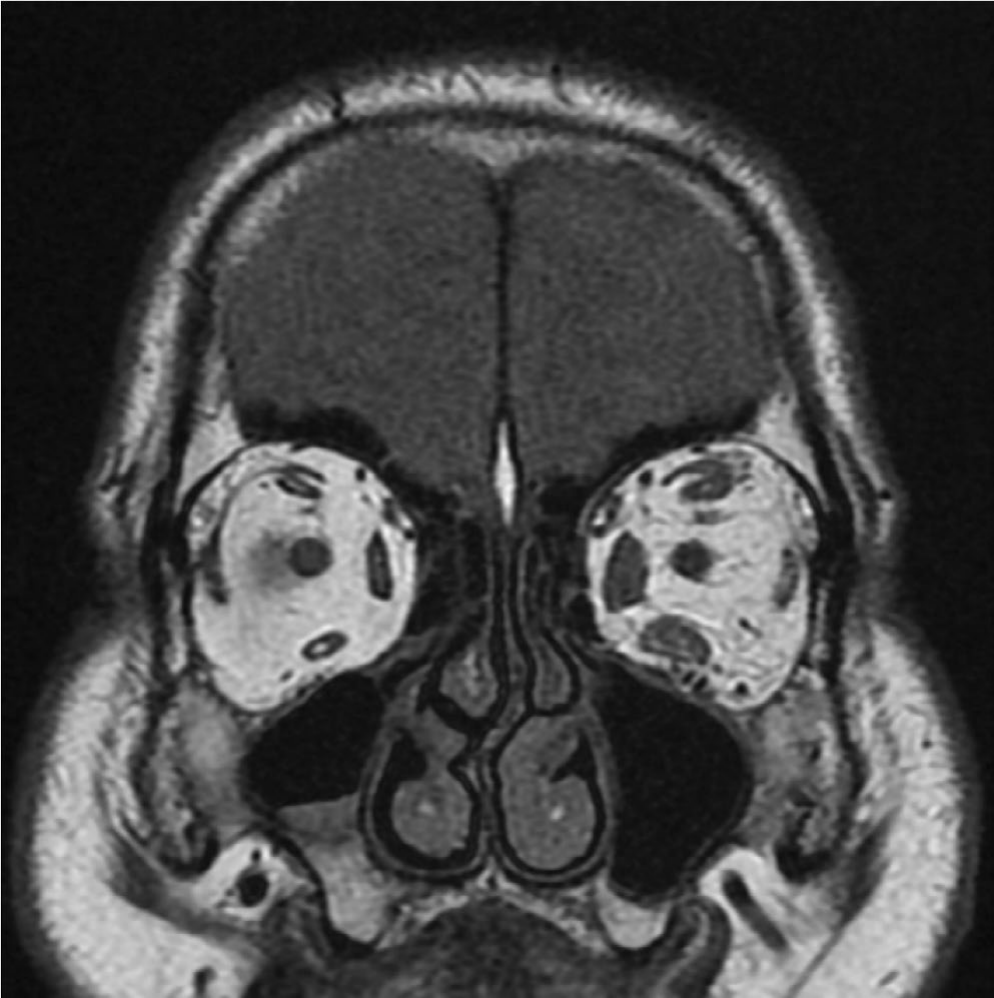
FIGURE 2 Orbital MRI demonstrated thickening of the medial and inferior rectus muscles in the left orbit
